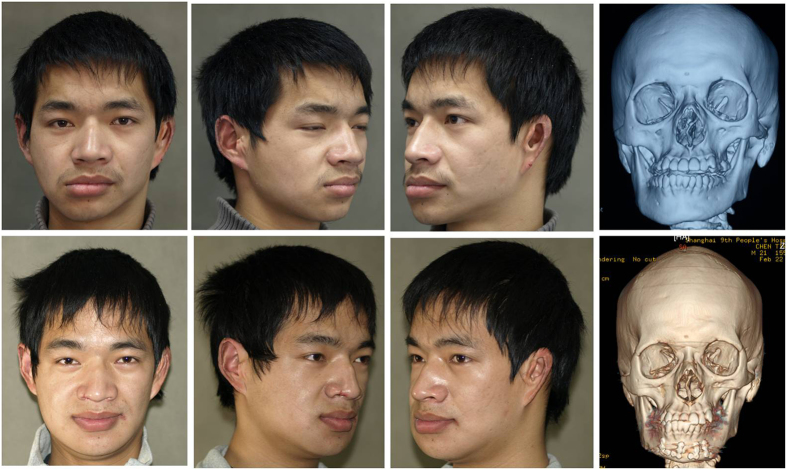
Photographs and computed tomographic (CT) scans before surgery (above) and three months after surgery (below) revealed a significant improvement in the facial symmetry.
